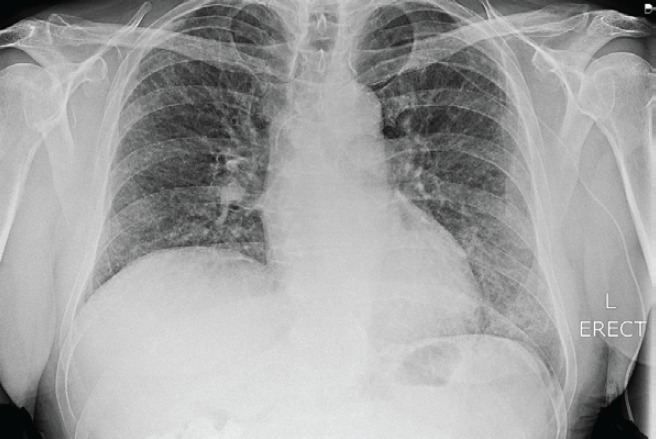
Panitumumab-induced fatal ILD. Chest X-ray on day 1 of admission, showing mild interstitial shadowing in both lungs.
